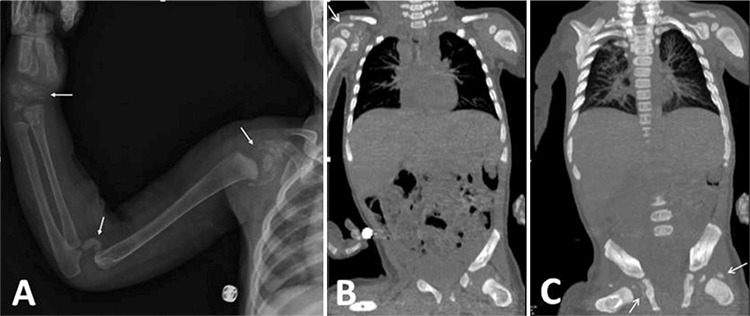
At age nine months, radiograph before magnesium and anti-phosphate treatment, A) Radiograph shows progression of periarticular calcification in the right shoulder, elbow and wrist joint, B) Coronal non-contrast computed tomography of abdomen demonstrates periarticular calcification in the right shoulder joint, and C) shows periarticular calcification in right hip joint
